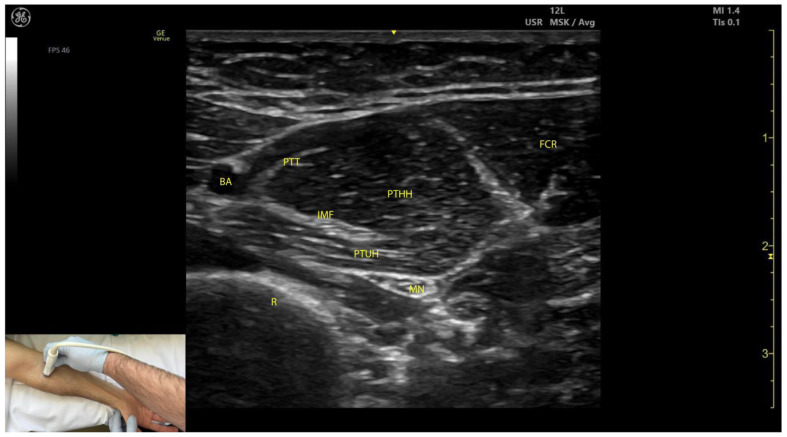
Ultrasound anatomy of the pronator teres (PT) with key landmarks: BA—brachial artery; PT—pronator teres; PTT—pronator teres tendon; R—radius; MN—median nerve; IMF—intramuscular fascia; PTUH—pronator teres ulnar head; PTHH—pronator teres humeral head; FCR—flexor carpi radialis.
